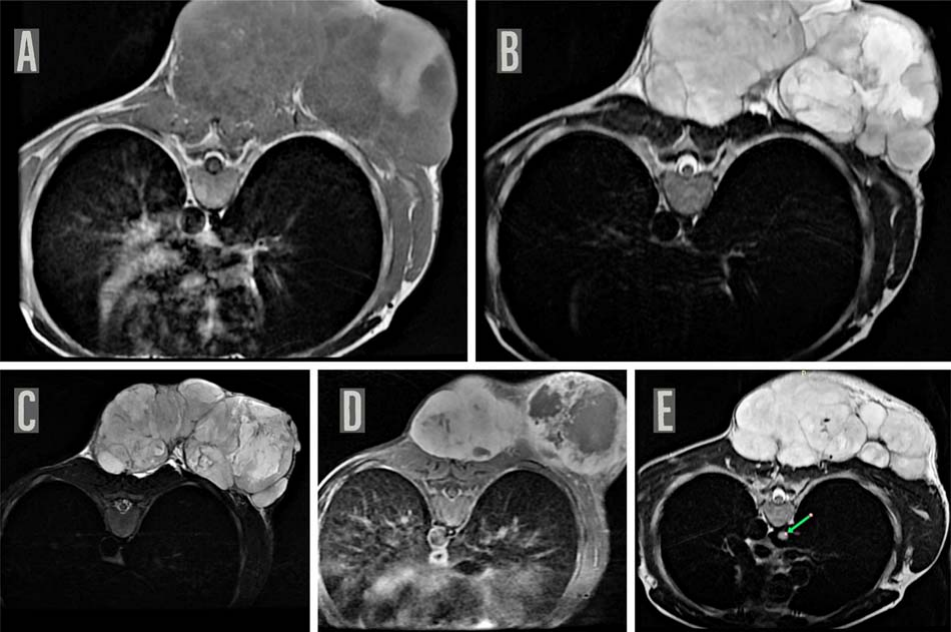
MRI chest. MRI of the chest on axial sections reveal a large well defined lobulated soft-tissue mass in the subcutaneous plane of the posterior chest wall, predominantly iso-hypointense on T1WI (A) with few areas of T1 hyperintensity and hyperintense on T2W image (B) and STIR images (C) and showing heterogenous enhancement with few central non-enhancing areas of necrosis on post contrast T1W images (D). Multiple internal septations were noted within the lesion with significant external contour bulge abutting the posterior chest wall muscles as well as bilateral posterior paraspinal muscles suggestive of a neoplastic process, possibly a mesenchymal tumor. MRI chest also shows a suspicious nodule (8 mm maximum diameter) appearing hyperintense on T2W image in the azygous lobe of right lung (indicated by green arrow) (E).
